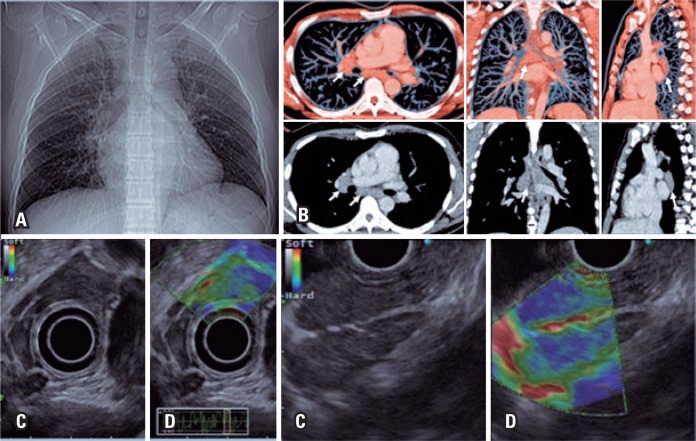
Mediastinal enlargement. (A) Chest radiograph; (B) Reconstruction of chest tomography in axial, coronal and sagittal cuts, with increased lymph nodes (white arrows); (C) Endoscopic ultrasound showed hypoechogenic, homogenous, lymph nodes measuring around 25mm; (D) Qualitative elastography with benign characteristics (green-like)
